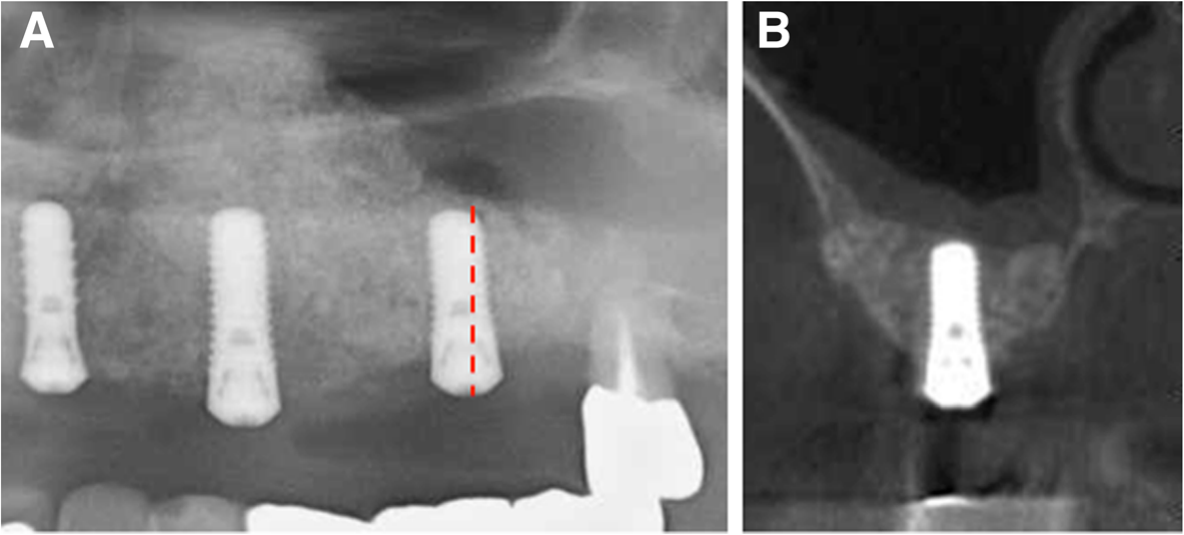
Radiographic image. Four months following 2nd sinus floor augmentation with implant placements. a Significant bone augmentation is achieved in site #26. b Coronal CT image of the *red line* shows that sufficient bone support with improved radiopacity was achieved in the palatal aspect of sinus cavity
